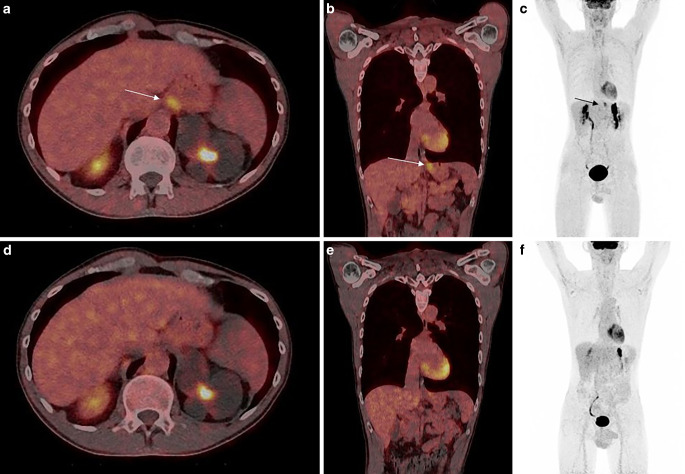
Transverse (a) and (b) coronal section of a fused dual-tracer [^18^F]FDG&[^68^Ga]Ga-FAPI-46-PET/CT and maximum intensity projection (*MIP*) in (c) show mild tracer uptake at the gastroesophageal junction, marked with *white* and *black arrows*. No tracer accumulation could be visualized in the same anatomic region after single-tracer PET/CT using [^18^F]FDG in fused images viewed in the axial (d) or coronal plane (e) or on MIP (f). Physiologic enhancement of the brain, myocardial, and urogenital systems is visible in both scans
